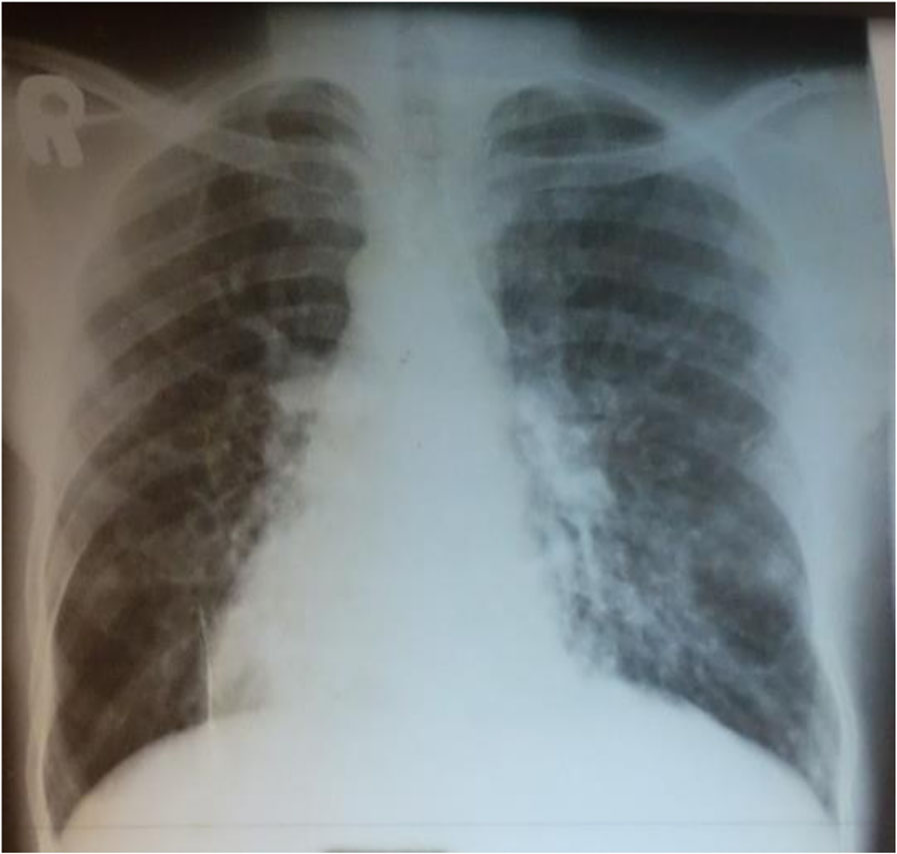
Posteroanterior chest X-ray showing dextrocardia with right-sided aortic arch. There is paracardiac and left lower lung bronchiectasis with fibrotic bands
